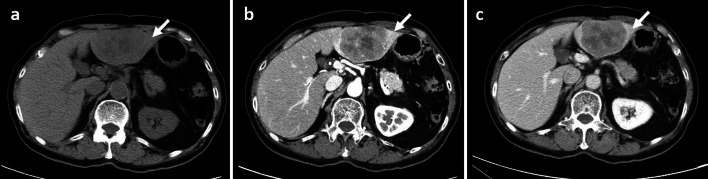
a–c Contrast-enhanced computed tomography (CECT): a plane; b arterial phase; and c portal venous phase. CECT showing a large well-defined and low-density mass with a diameter of 66 × 55 mm in the left lateral segment of the liver. The mass appears to have a lower density than the surrounding liver tissue on plane computed tomography images (a) and enhanced heterogeneously (b). No ascites or enlarged lymph nodes were detected
